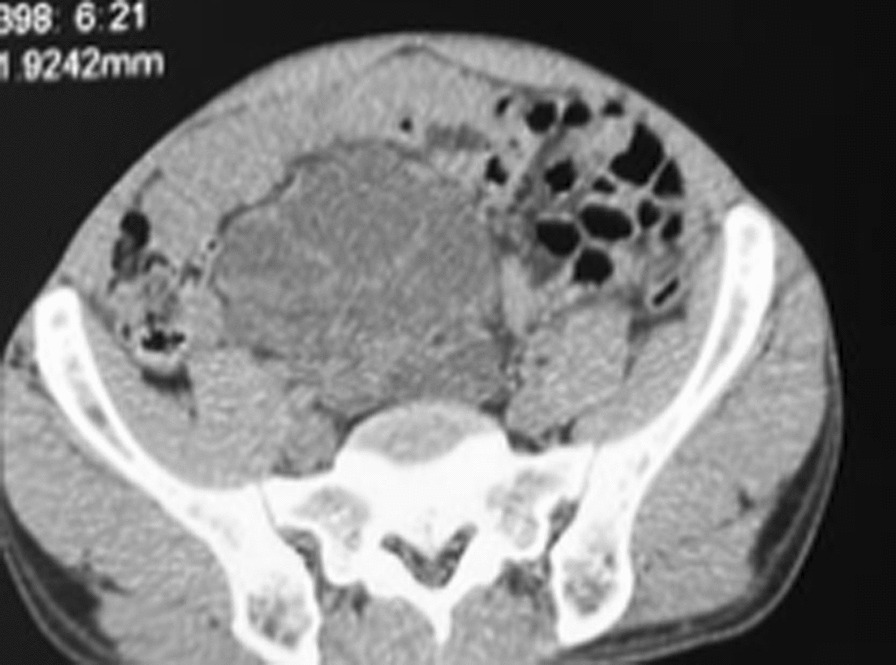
Right ectopic polycystic kidney disease on CT—Scan (transverse view)
